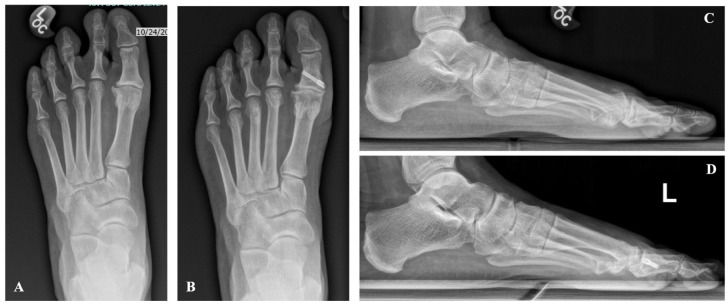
Weightbearing radiographs of the left foot. (A) Preoperative anteroposterior view. (B) Six-month postoperative anteroposterior view. (C) Preoperative lateral view. (D) Six-month postoperative lateral view. Images demonstrate radiographic outcomes following Moberg osteotomy and cheilectomy.
